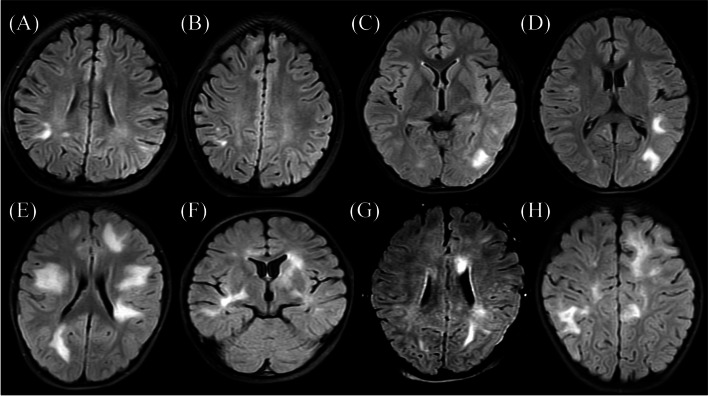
Brain MRI findings of patients with MOG-Ab-positive ADS (A–H)
